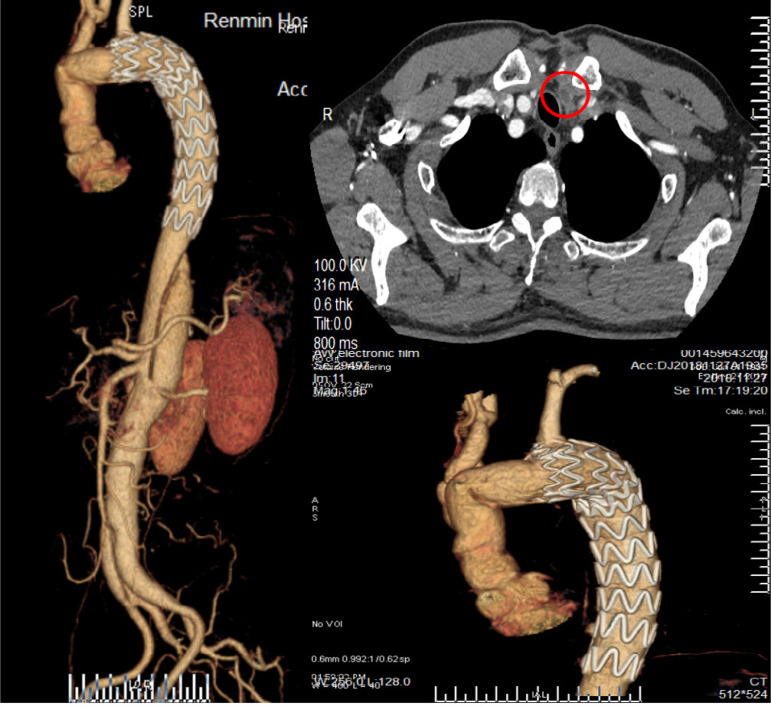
Computed tomography angiography following total arch replacement in Case 2 showed normal development of brachiocephalic trunk and left subclavian artery but no development of the left common carotid artery.
